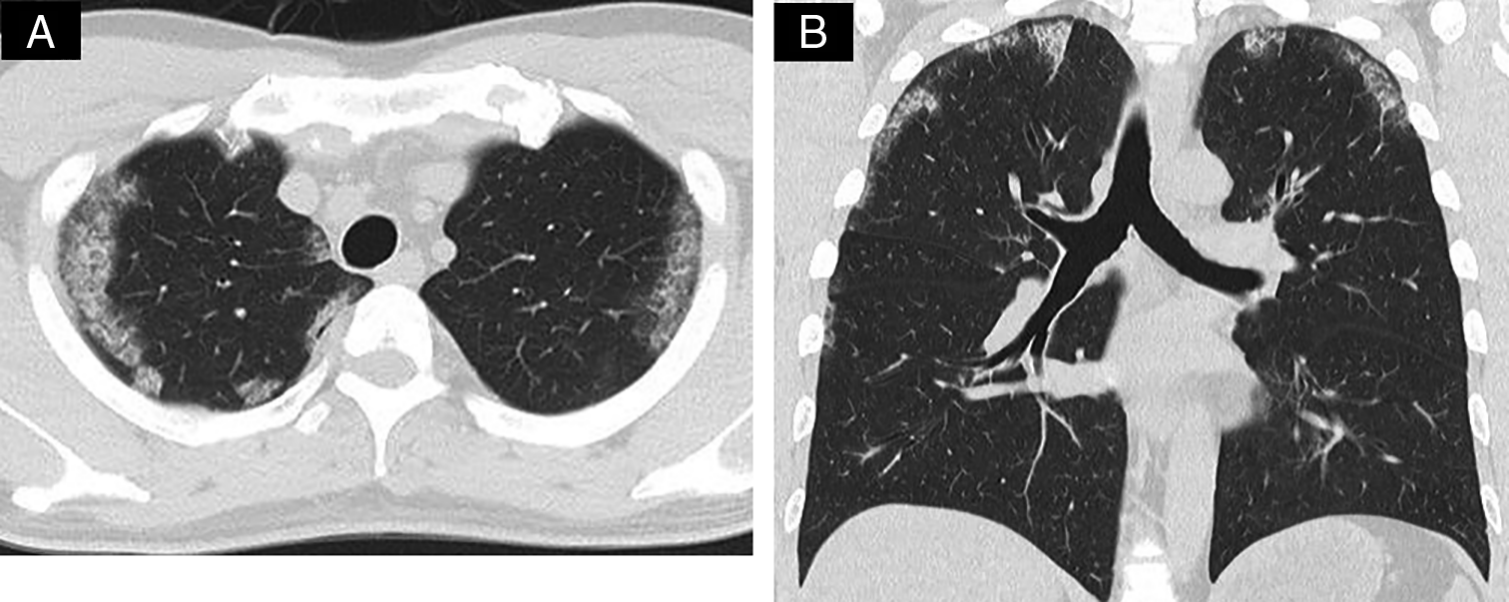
Chest computed tomography (CT) images demonstrates peripheral ground‐glass opacities in bilateral upper lobes. (A) Transverse section on chest HRCT, (B) coronal images of chest CT.
